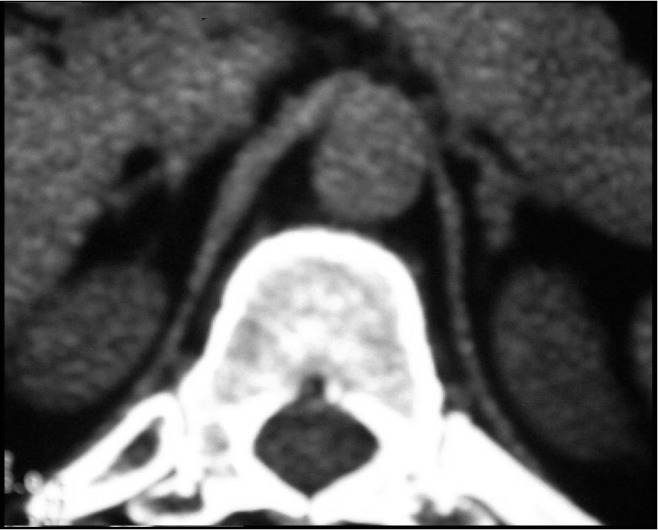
Adrenal computed tomography scan of the adrenals of a patient with Carney complex.
